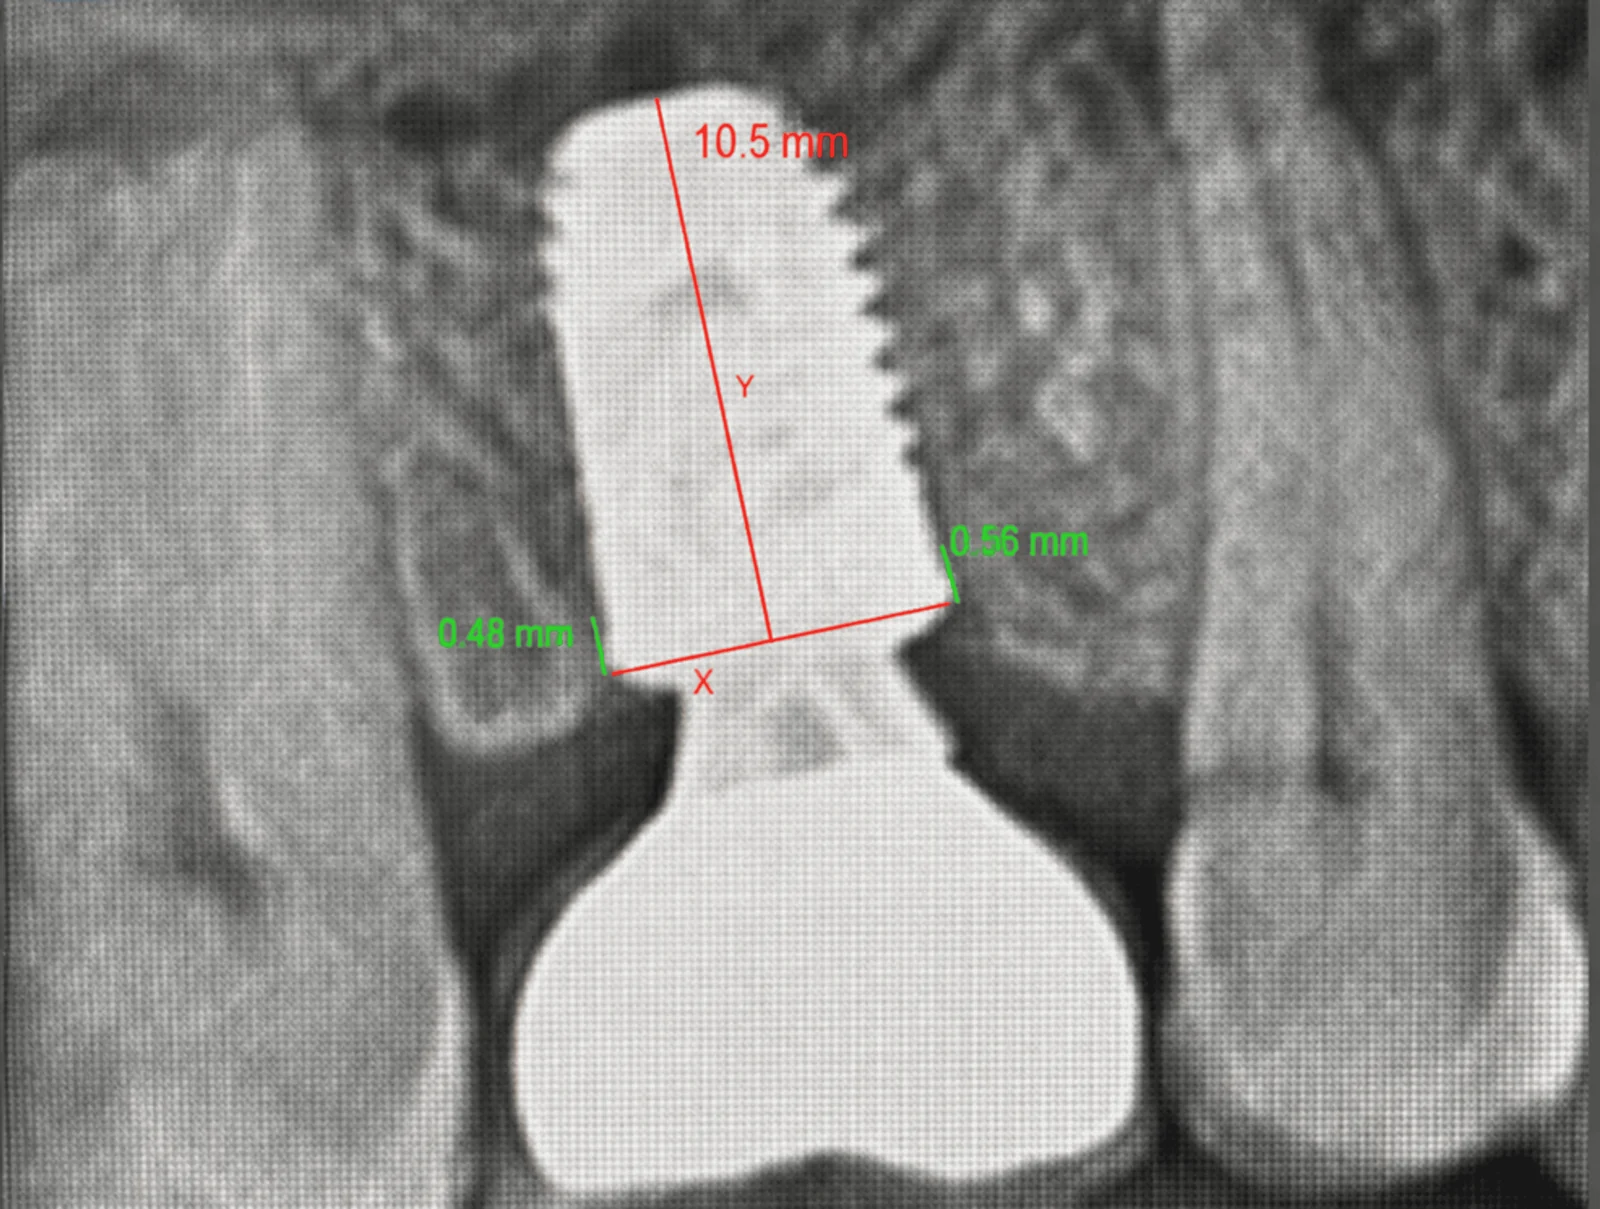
Measurement of radiographic bone loss on mesial and distal aspects of maxillary posterior implant. Green line: distance from implant platform to nearest apical location of bone to implant surface contact; red line (X): implant platform; red line (Y): implant length.
